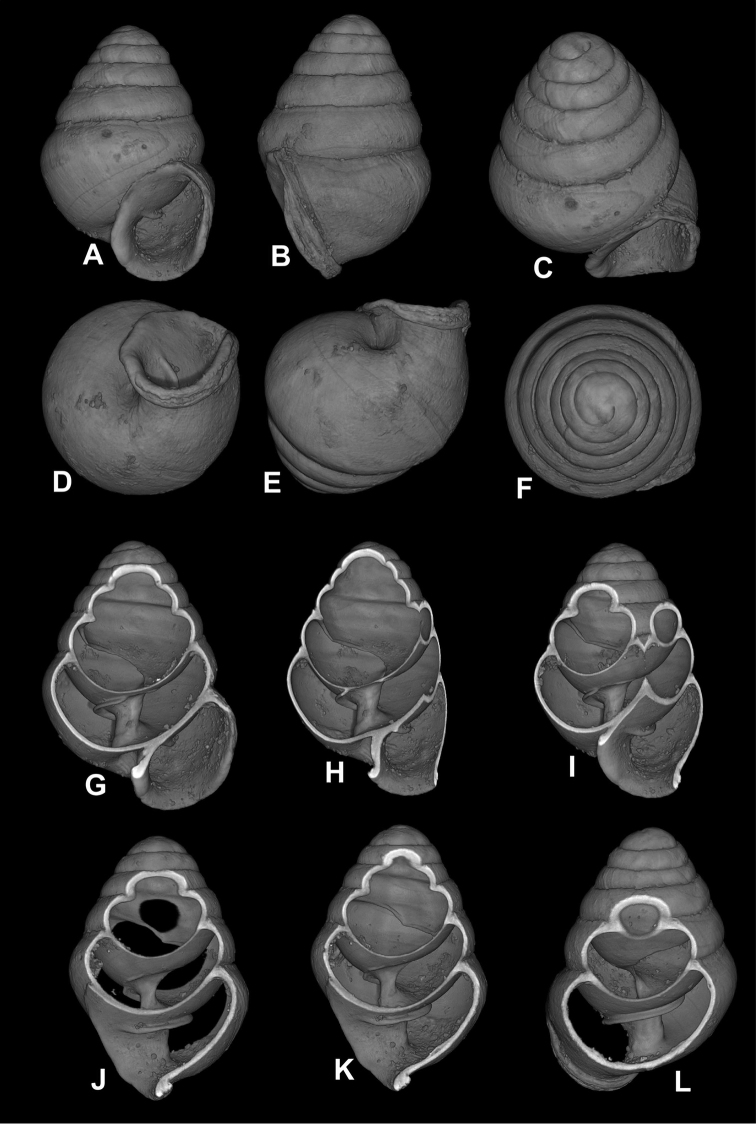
Nano-CT images of *Koreozospeum
